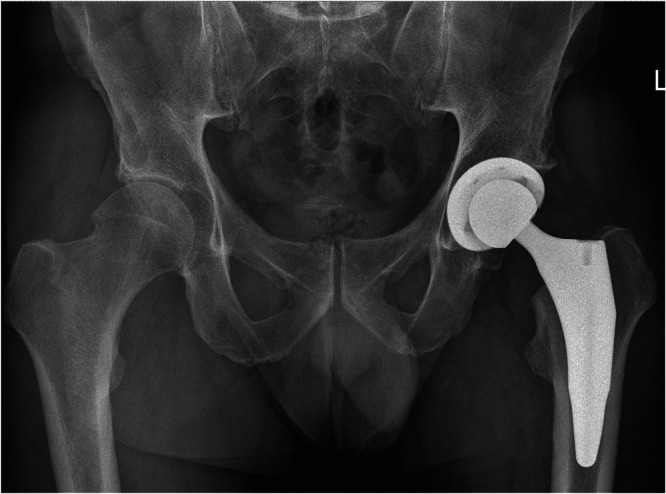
Two‐year postoperative radiographic follow‐up of a lateralizing 3C cementless short femoral stem (CCD angle: 127.5°). CCD, Caput–Collum–Diaphyseal.
